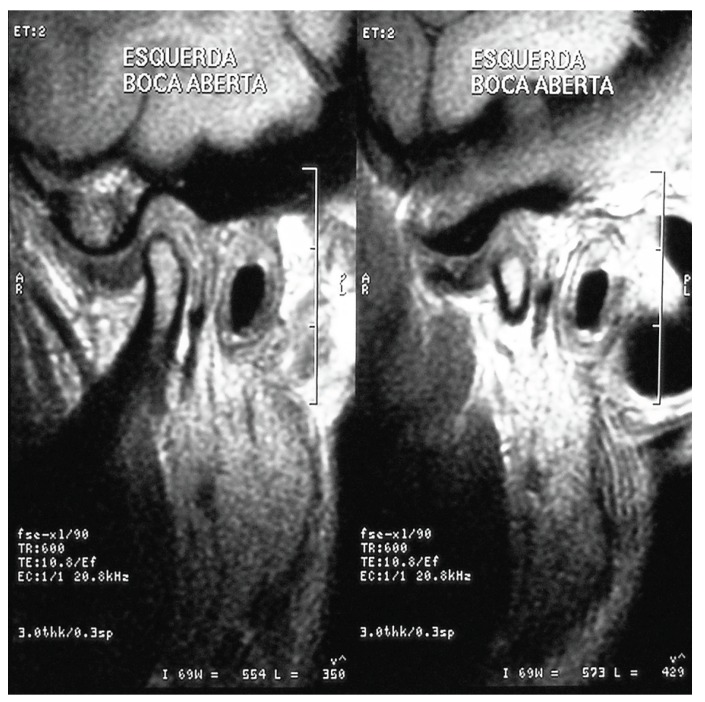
MRI findings of Anterior disc displacement without reduction.
